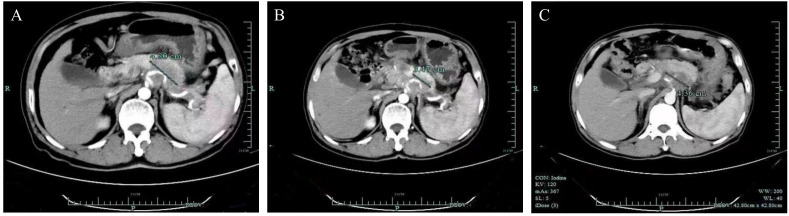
Computed tomography scans of the targeted lesion. (A) Baseline. (B) After three doses of pembrolizumab, demonstrating a partial response (PR) of the lesion in the pancreas. (C) After six doses of treatment, the lesion was enlarged.
